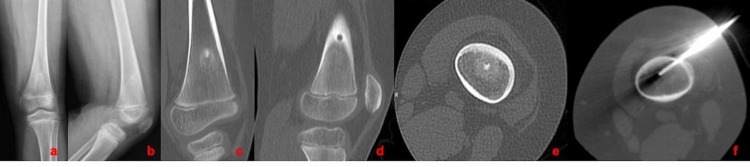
A nine-year-old male patient with OO localized in a) the femur; b) AP and lateral X-ray; c), d) and e) coronal, sagittal, and axial CT view; and f) axial CT view during the RFA procedure OO: osteoid osteoma; AP: anteroposterior; CT: computed tomography; RFA: radiofrequency ablation
